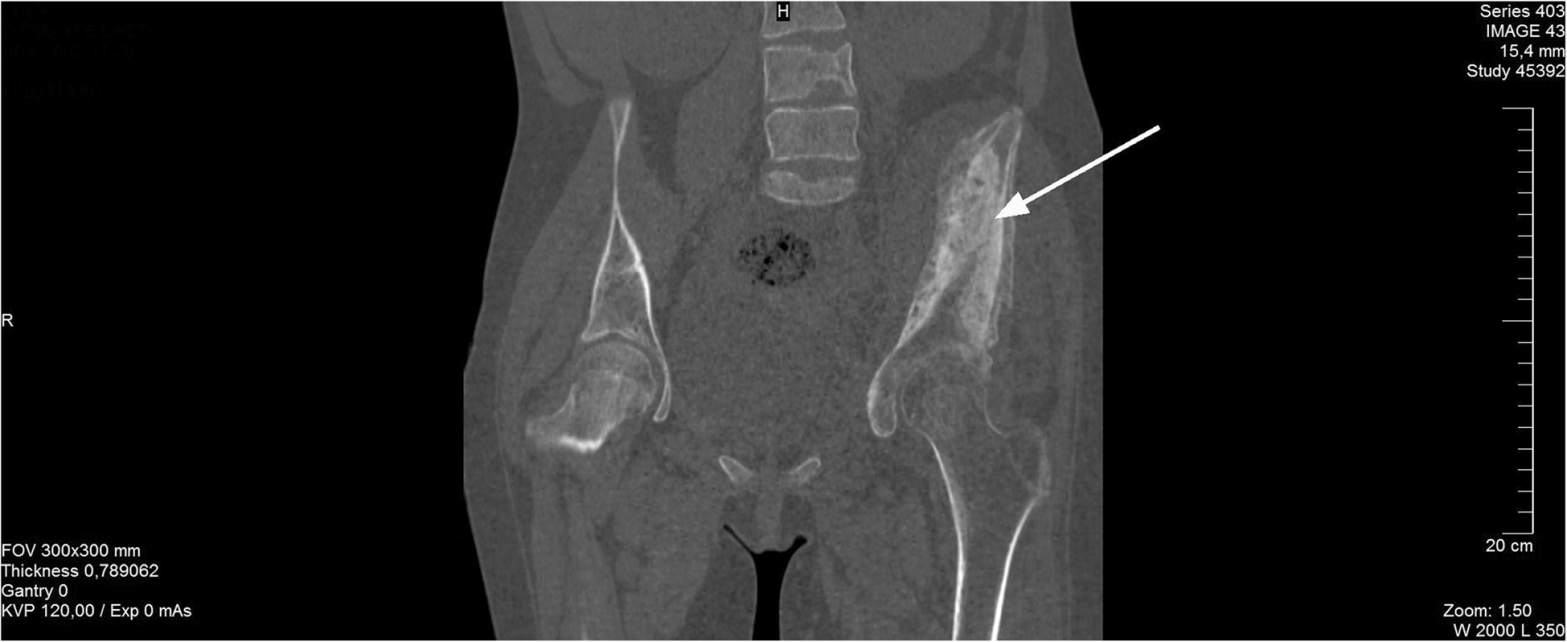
Whole-body CT (pelvis region) showing destruction and sclerosis of the left hip bones
